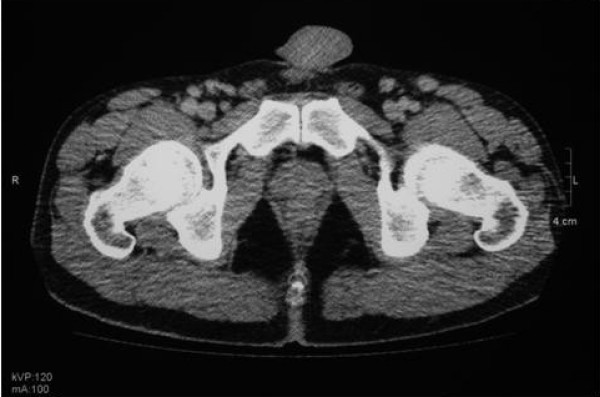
CT scan of pelvis indicating multiple enlarged lymph nodes.
